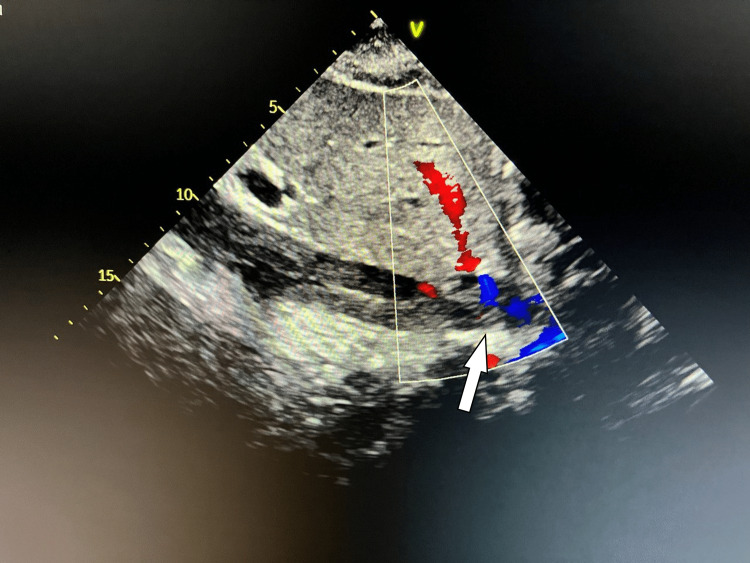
Color doppler echocardiography (ECHO) showing dilation and near total occlusion of the inferior vena cava (white arrow) with considerably reduced flow.
